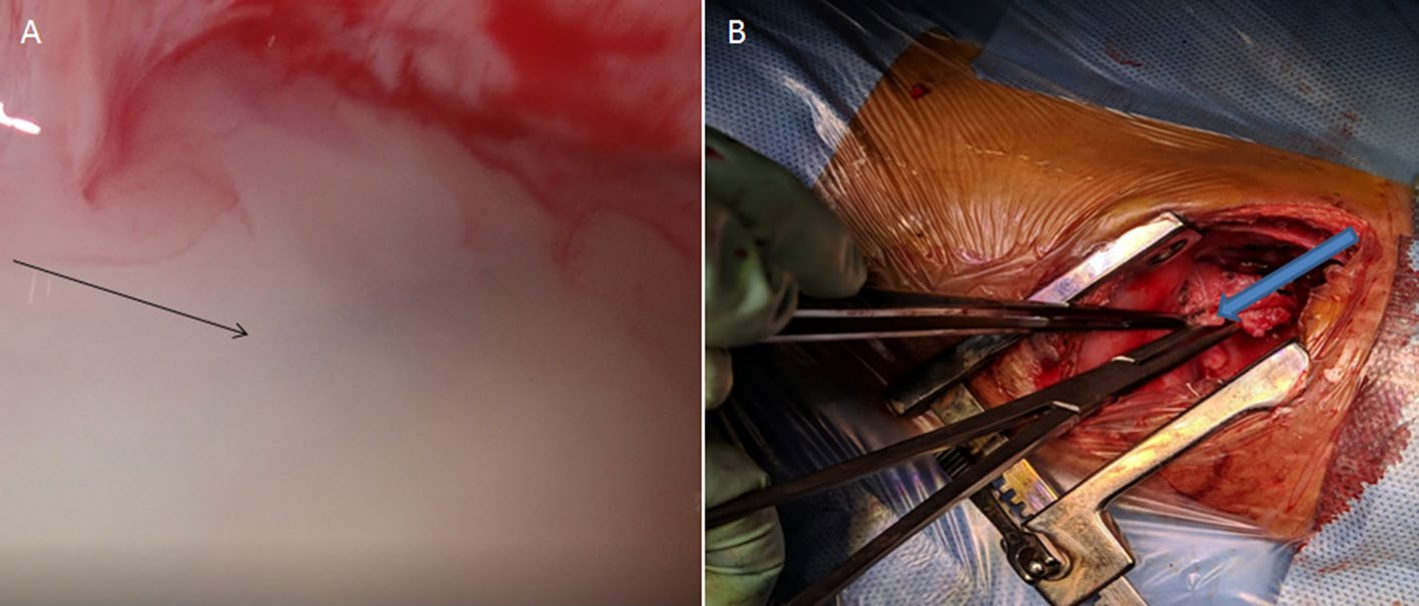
Thoracoscopic/open en masse ligation of thoracic duct surgery performed after [99mTc]sulphur colloid lymphoscintigraphy.(A)Intraoperative images during thoracoscopic surgery showed frank chylous fluid (as shown by black arrow). There was difficulty in identification of thoracic duct from esophagus and aorta during thoracoscopic surgery so procedure converted to open and en masse ligation. B Thoracic duct held by the artery forceps (as shown by blue block arrow). After surgery medium chain triglycerides were started on post-operative day 5
